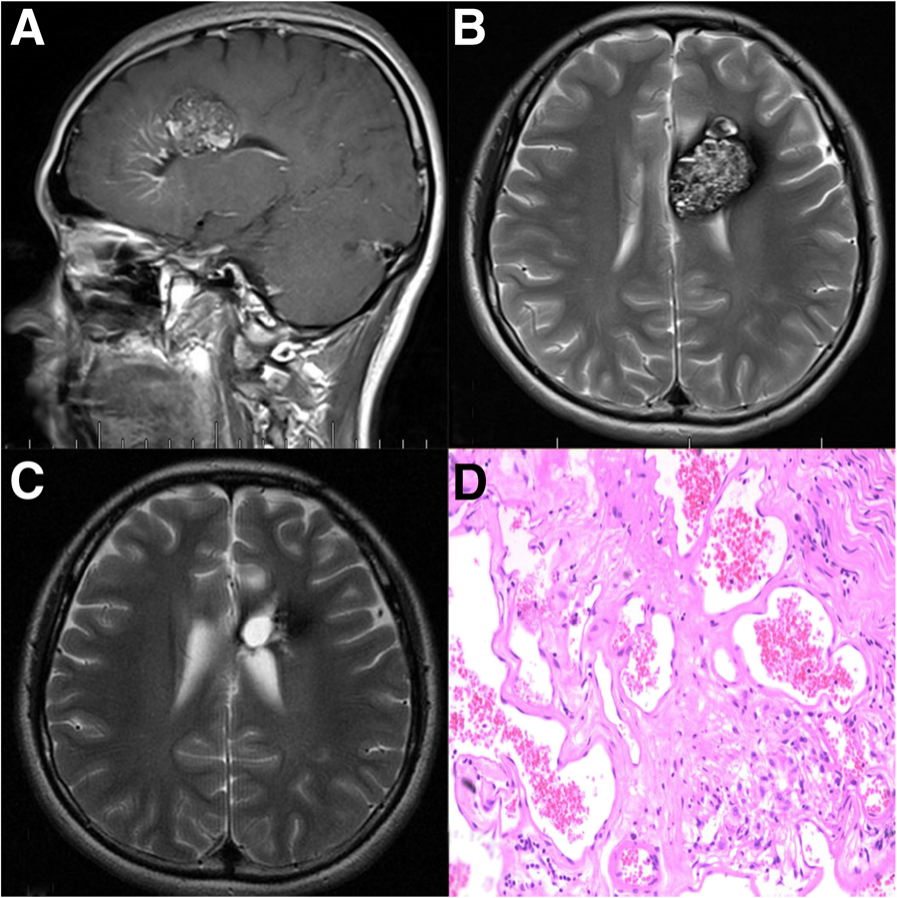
Supratentorial DVA and Concomitant CM. A: Enhanced MRI image showing the DVA and the CM which locates at the distal radicles of the DVA. B: CM shows classic heterogeneous “popcorn” appearance on T2-weighted image. C: Post-operation image showing that the CM disappeared. D: Pathological picture of the CM showing the sinusoidal vascular channels.
